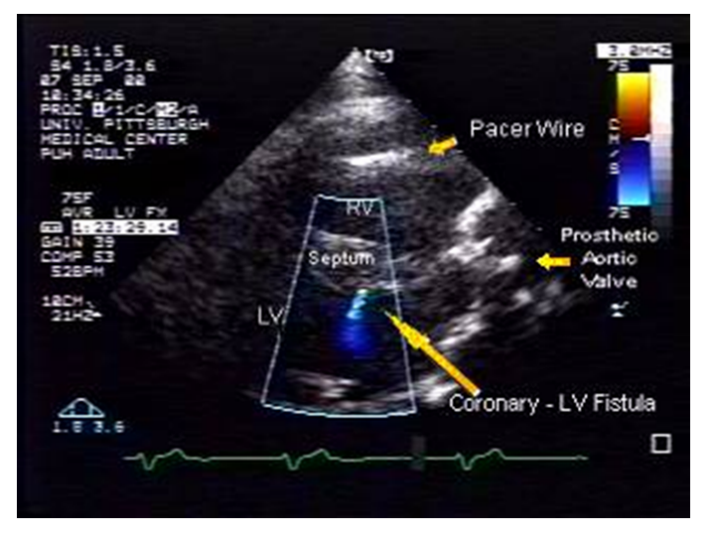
Similar parasternal image showing the predominantly diastolic color flow image component. (RV = right ventricle, LV = left ventricle, LA = left atria).
